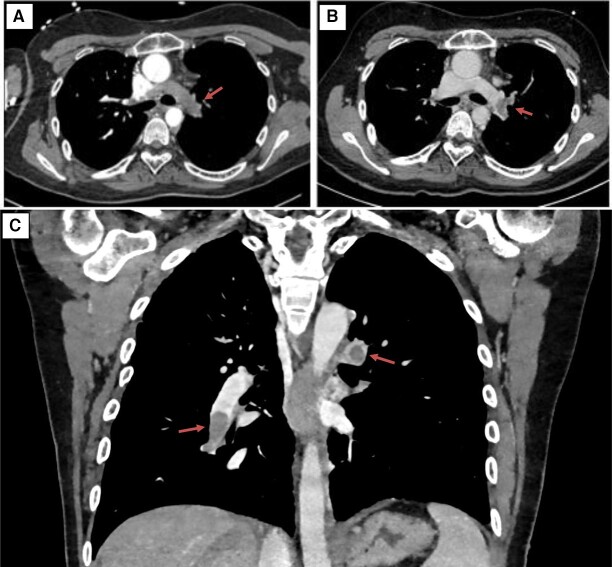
(*A* and *B*) Comparison between chest computed tomography performed without (left) and with (right) Fontan protocol. In both images, pulmonary thromboembolism is present (arrows). (*C*) A coronal view of the bilateral pulmonary thromboembolism (arrows).
